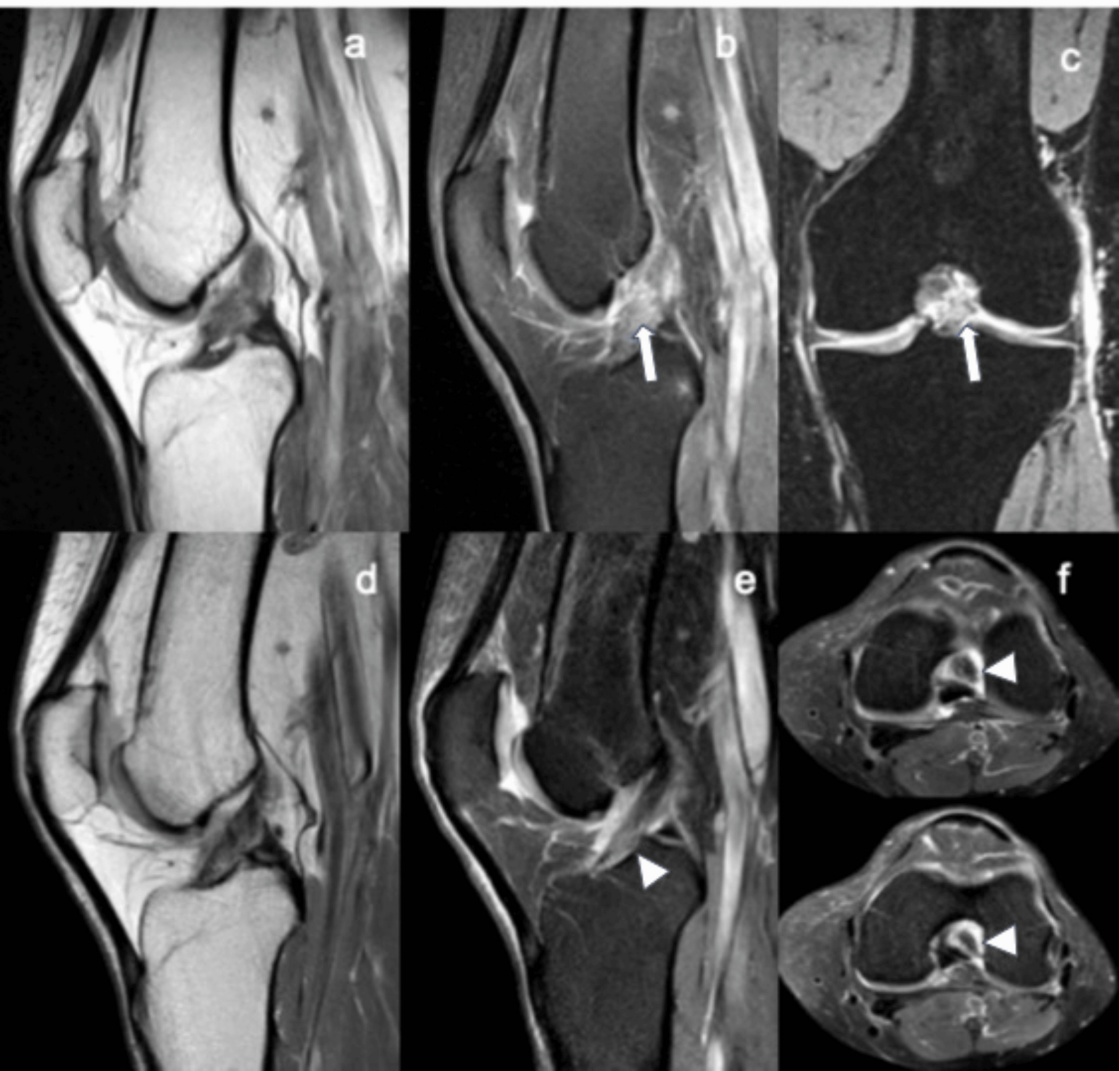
Magnetic resonance imaging eight days after knee trauma and after 24 weeks of conservative biological treatment. Case 5: magnetic resonance imaging baseline at eight days after trauma shows grade II-III anterior cruciate ligament rupture (white arrows) and grade I medial collateral ligament injury (a-c). Follow-up at 24 weeks post-ACS shows continuous anterior cruciate ligament (arrowhead) with high signal in the lower third (repair grade 1) (d-f). ACS: autologous conditioned serum
